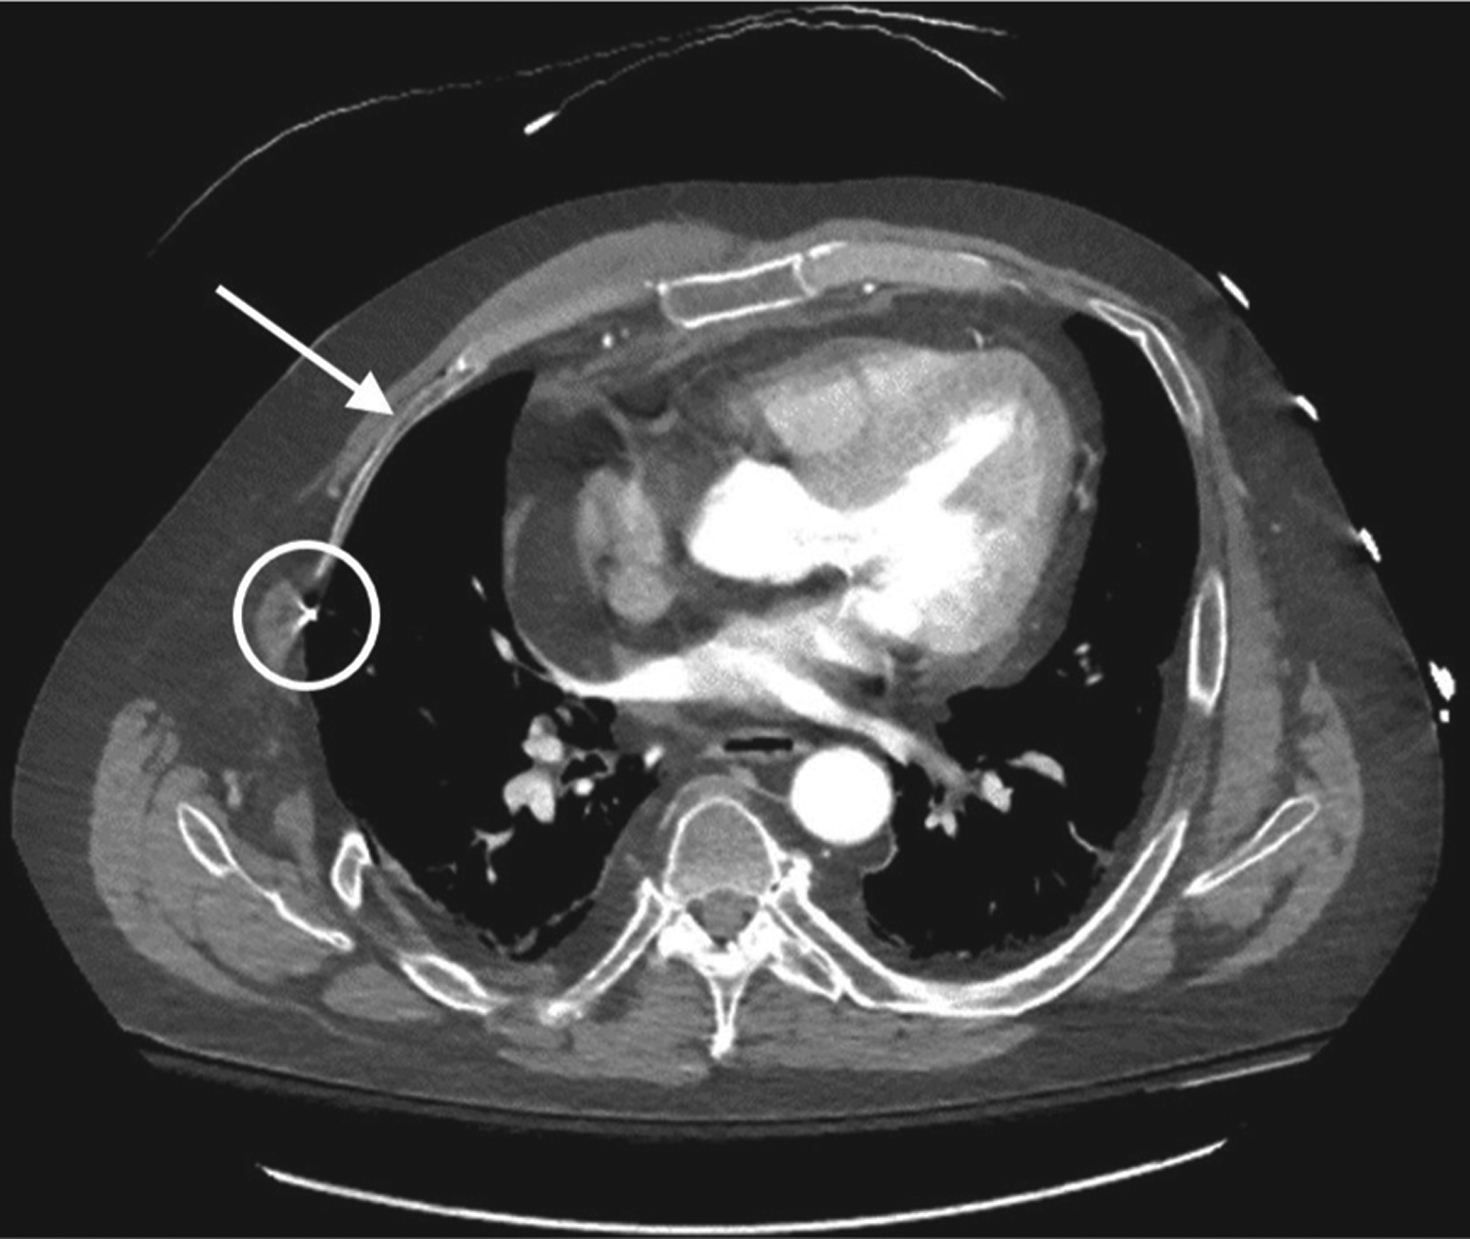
Computed tomography imaging of the chest showing clips (circle) from the patient’s previous right partial rib resection of ribs 3 and 4. Osteoporosis of the ribs is appreciated by the thinning of cortical bones (arrow)
